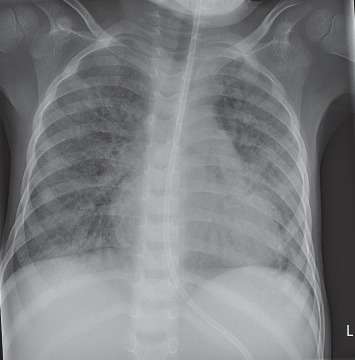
Chest X-ray after extubation.
